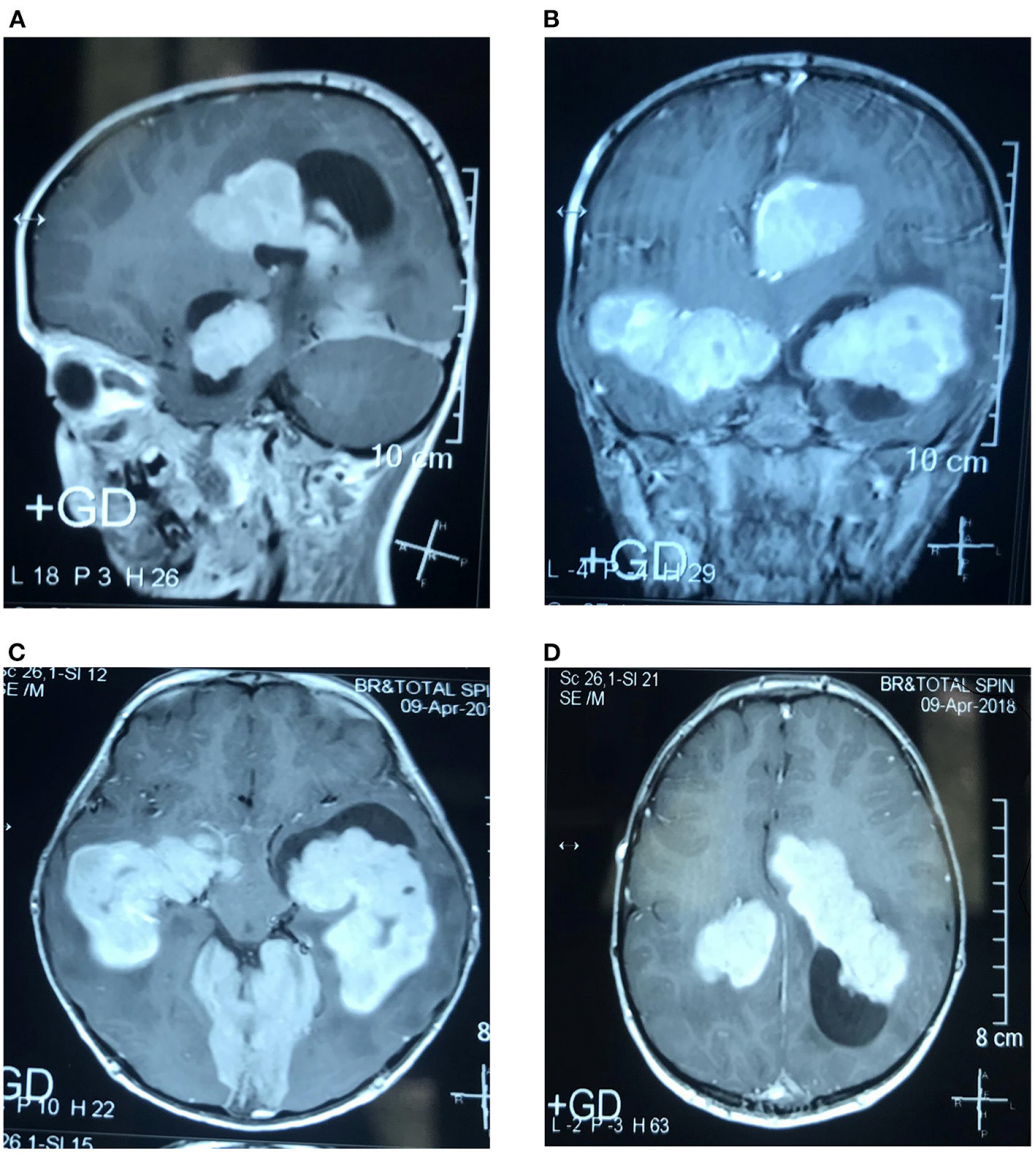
Sagittal (A), coronal (B), and axial (C,D). T1-weighted (T1W) contrast-enhanced MRI show multiple, homogeneously enhancing intraventricular masses.
